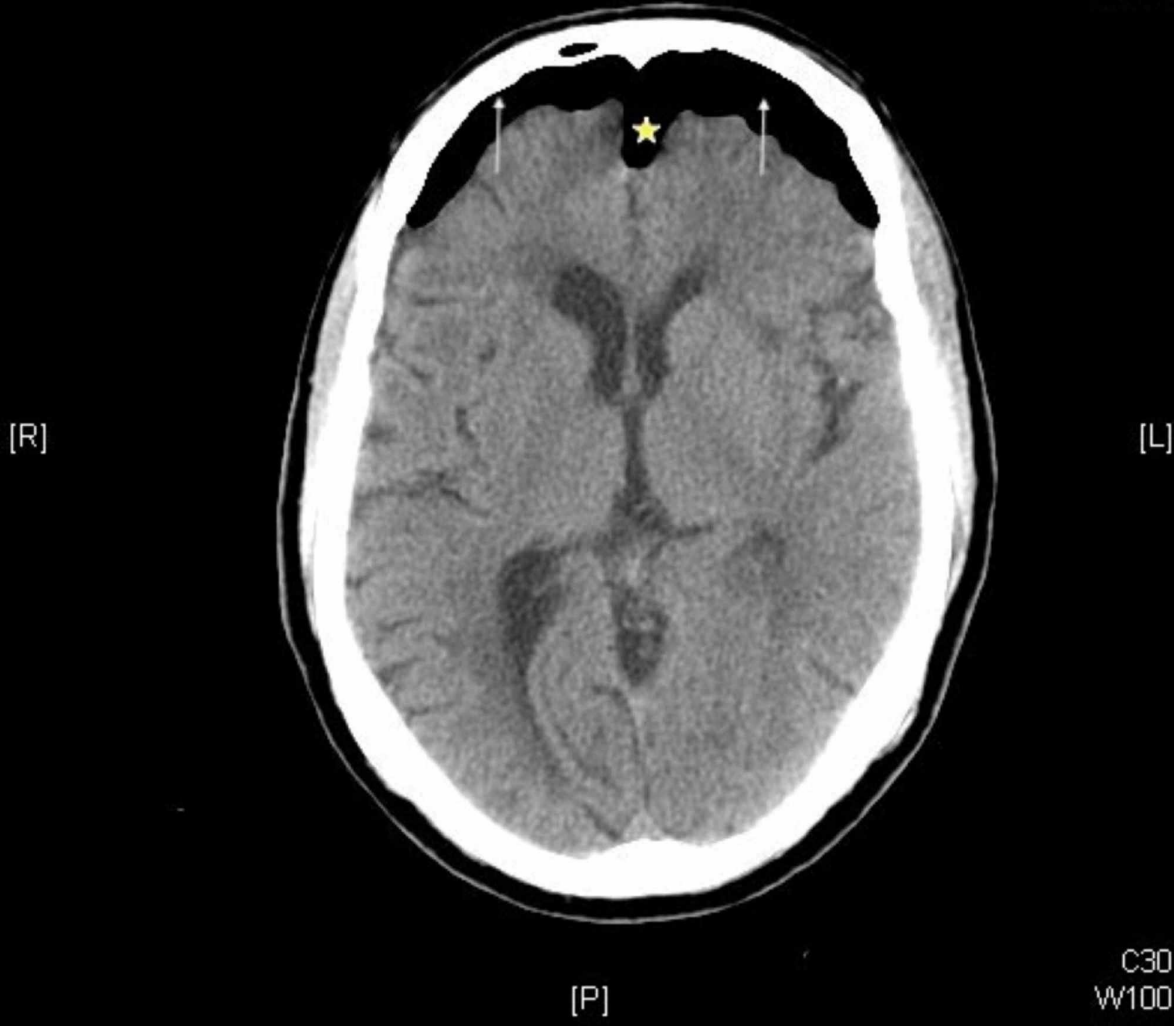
Noncontrast brain CT demonstrates subdural air (arrows). There is separation of the brain parenchyma from the falx cerebri, classically referred to as the Mount Fuji sign (star).
